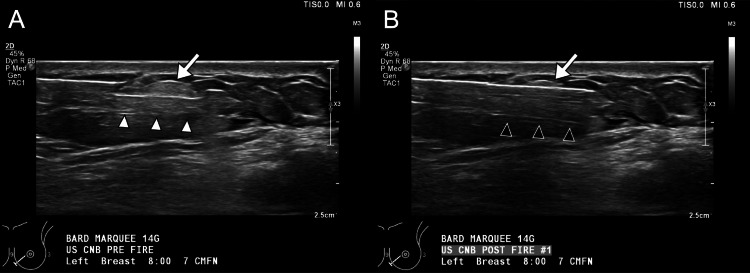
Ultrasound-guided core needle biopsy of the superficial mass in the left breast at the contact zone. (A) Pre-fire and (B) post-fire grayscale images show successful placement (white arrowheads) and deployment (black arrowheads) of core needle biopsy of the mass (arrow).
